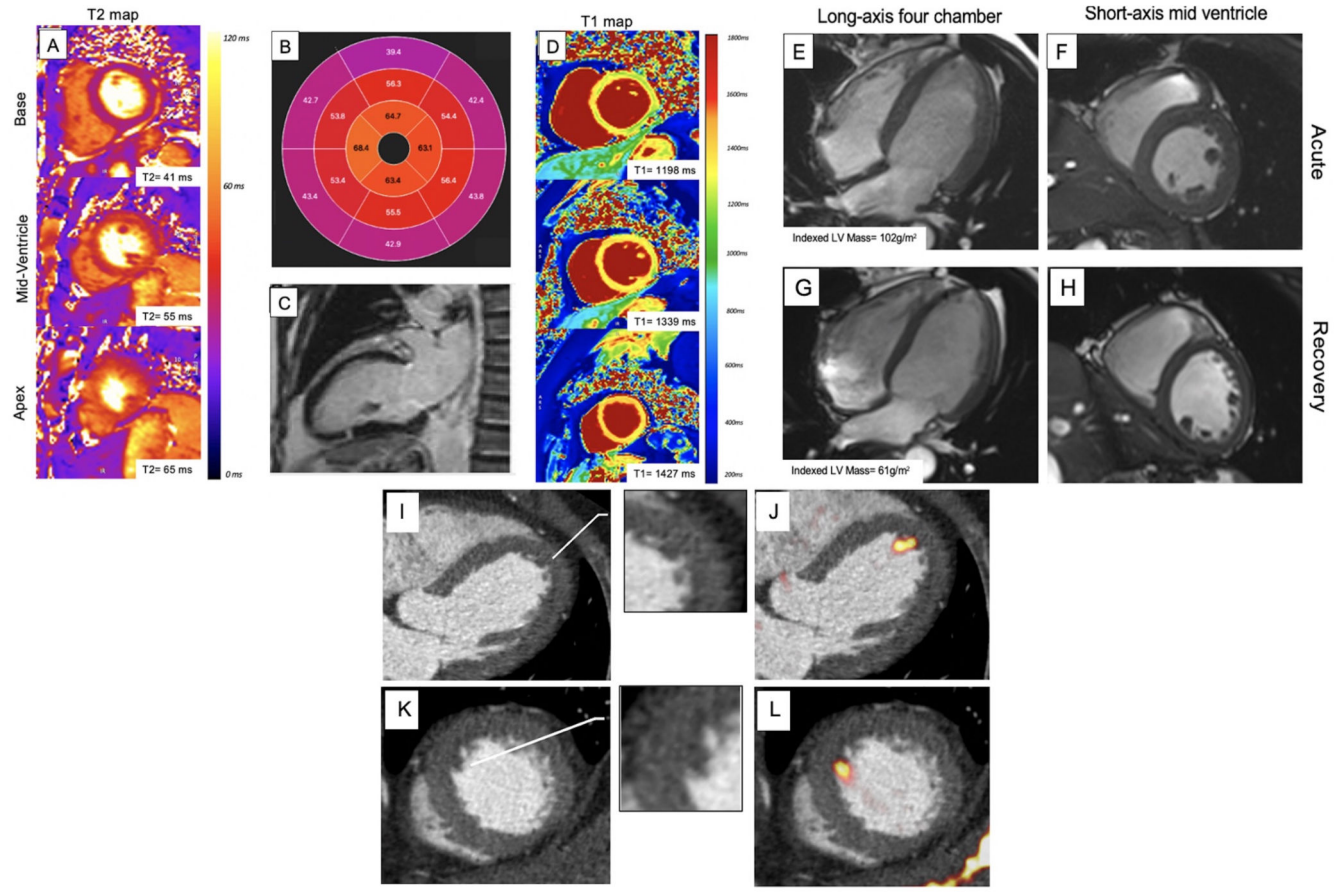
Cardiac Magnetic Resonance Imaging and Computed Tomography Findings in Takotsubo Syndrome Short axis T2 maps (A), T2 polar map (B) and short-axis T1 maps (D), demonstrating elevated T2 and T1 values circumferentially in the mid and apical regions (outside of a coronary territory). Long axis two-chamber view demonstrating transmural fibrotic band pattern typical of takotsubo syndrome 4 months after the index event (C). Long axis four-chamber and short axis views of mid-ventricle demonstrating elevated left ventricular mass in acute phase (E, F) and normalization during convalescence (G, H). Hybrid positive emission tomography with cardiac computed tomography angiography depicting a small left ventricular thrombus in a patient with takotsubo syndrome with no clinically apparent thrombus on conventional imaging. There is subtle hypoattenuation on the computed tomography angiogram (magnified inserts) and increased uptake of an activated platelet and thrombus-specific radiotracer (^18^F-GP-1; yellow-red) in long axis four-chamber (I, J) and short axis (K, L) views on positron emission tomography.
